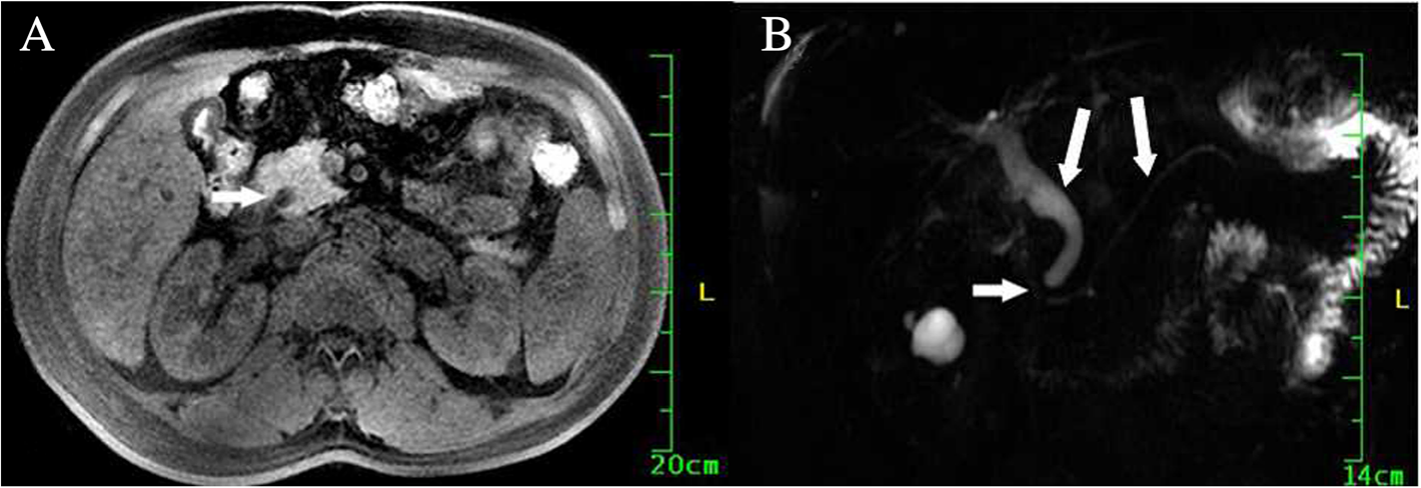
Magnetic resonance imaging findings on 22 February 2011. (A) Short inversion time inversion recovery image, showing a mildly dilated common bile duct. Heterogeneous isointense areas (white arrow) were observed in the distal portion of the common bile duct, suggesting common bile duct stones. (B) Magnetic resonance cholangiopancreatography, showing mild obstruction at the duodenal ampulla (short white arrow). The common bile duct and pancreatic duct were slightly dilated (long white arrows).
